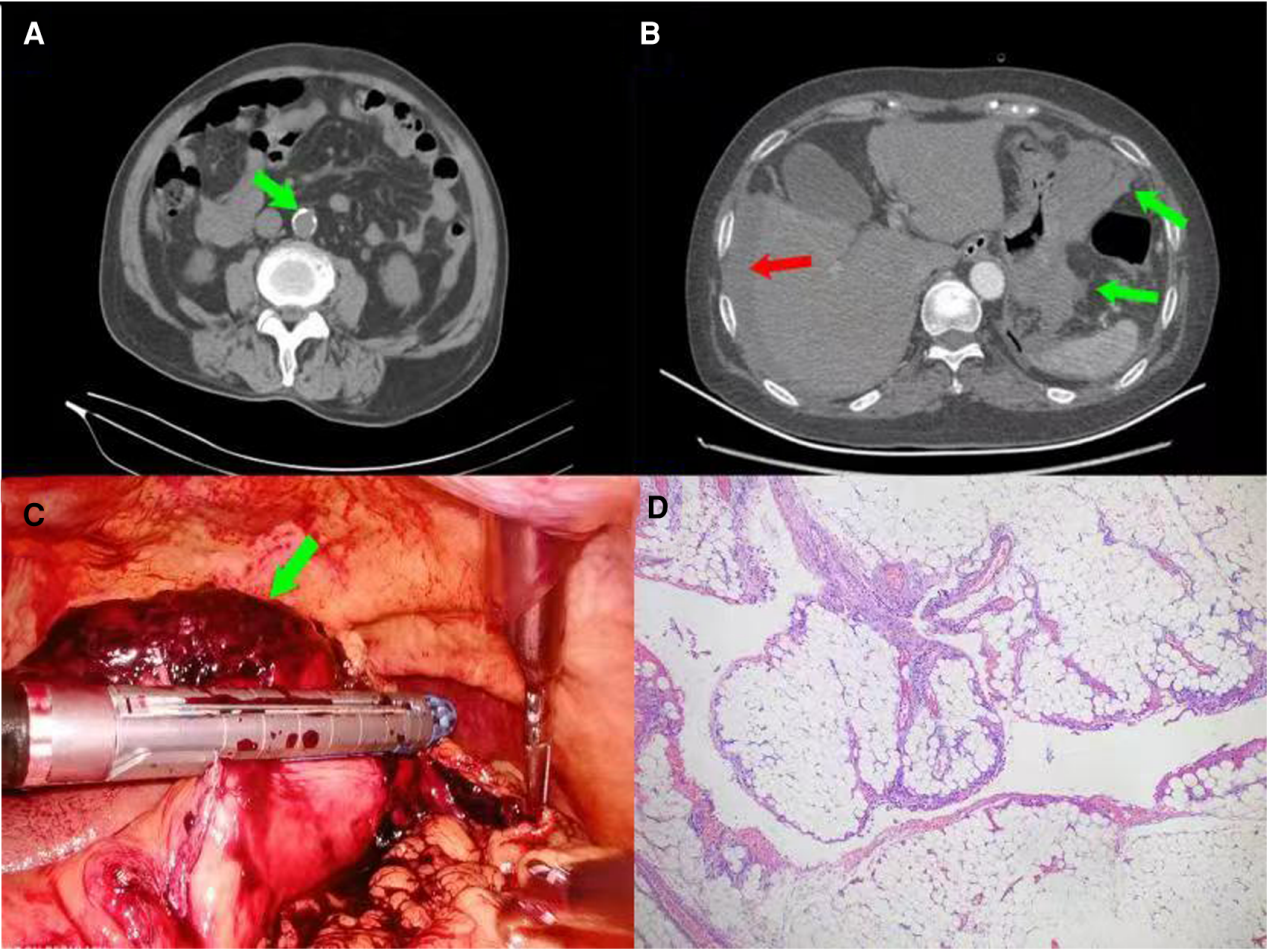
(Case 2) (A): CT-scan showing the formation of calcified plaques in the abdominal aorta (green arrow), bilateral common iliac artery, bilateral internal iliac artery, and right external iliac artery, partially accompanied by mural thrombosis; (B): enhanced CT of the upper abdomen showing hematoma formation in the upper left abdominal cavity, an unclear boundary between the hematoma and stomach (green arrows), and abdominal pelvic fluid/blood (red arrow). (C): The procedure was conducted by removing the mass and partly attached gastric wall with an endoscopic linear cutting stapler (EC60A, Ethicon). (D): Postoperative pathological examination showing a ruptured cavernous hemangioma with hemorrhage and thrombosis (HE × 100).
